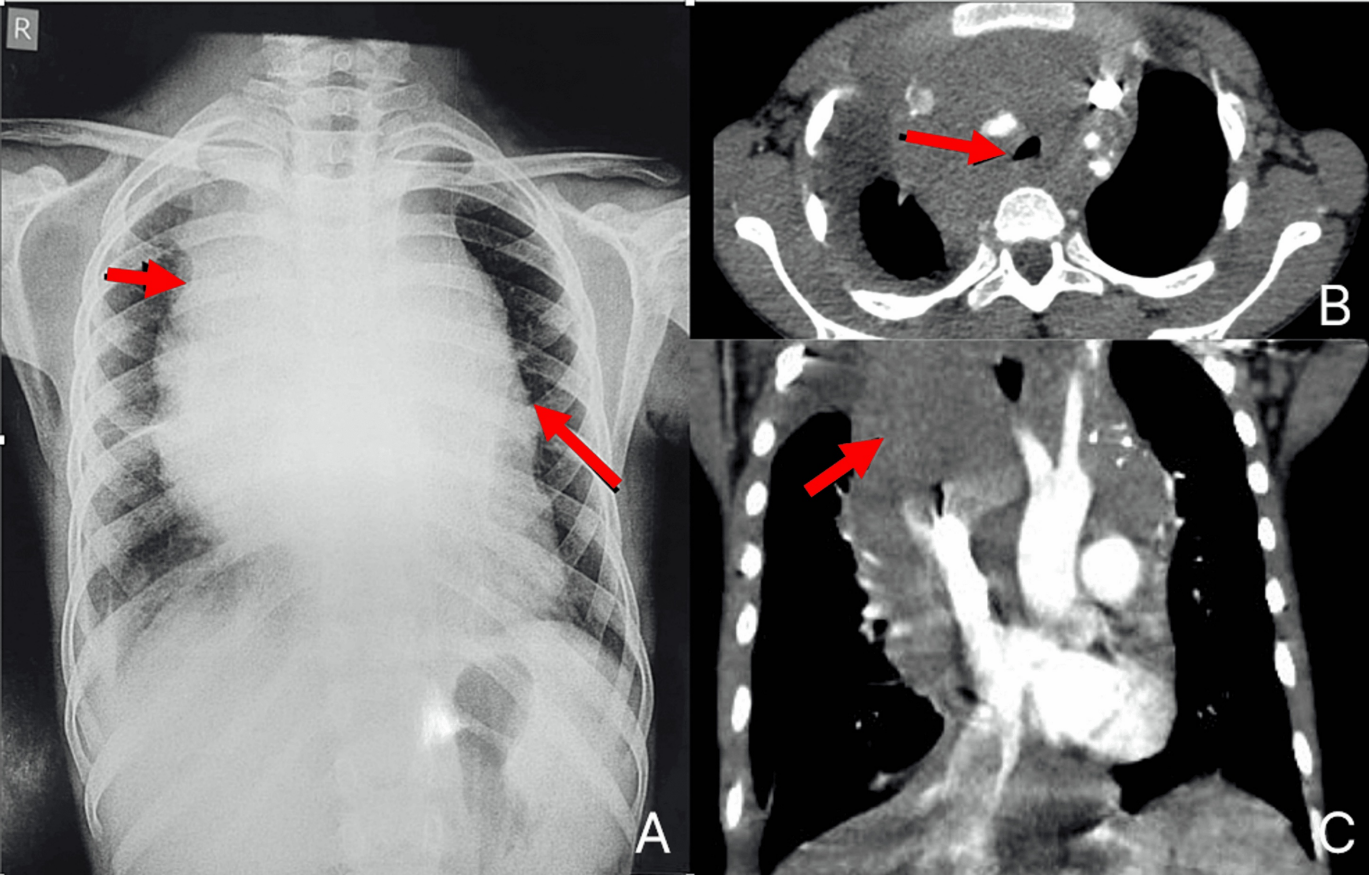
(A) Chest X-ray in PA view. (B) HRCT thorax axial section demonstrating tracheal deviation and compression. (C) HRCT contrast coronal section showing the extent of the mass. (A) Chest X-ray showing the mediastinal mass. (B) HRCT thorax axial section demonstrating tracheal compression by the mediastinal mass. (C) HRCT contrast coronal section revealing the mass, approximately 11.3 × 6 × 11.9 cm (CC × AP × TR), encasing the aorta, superior vena cava, both innominate veins, proximal bilateral subclavian arteries, left common carotid artery, brachiocephalic trunk, azygos vein, bilateral internal mammary vessels, and pulmonary arteries. The mass extends into the pre-tracheal, para-tracheal, retro-tracheal spaces, aortopulmonary window, and pre-vascular space, showing mild near-homogeneous enhancement with areas of necrosis. The superior vena cava is compressed anteroposteriorly and displaced posteriorly, while the azygos vein is displaced laterally. HRCT, high-resolution CT
